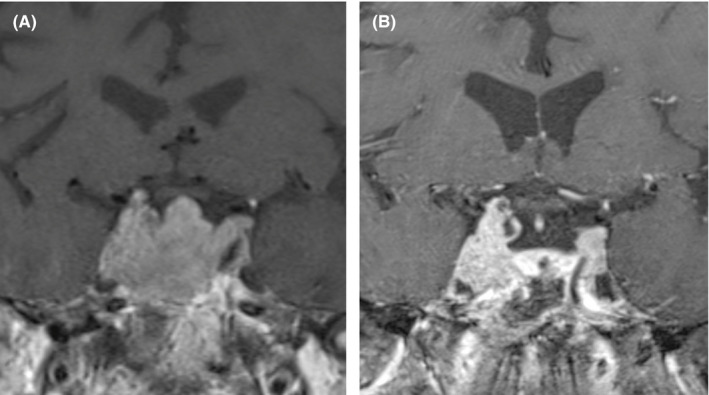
Pre‐ and post‐surgical MRI of the pituitary gland. (A) Post‐contrast coronal T1‐weighted MRI shows sellar mass invading the cavernous sinuses bilaterally. (B) Post‐contrast coronal T1‐weighted MRI after transsphenoidal resection shows persistence of residual tumor in the cavernous sinuses
